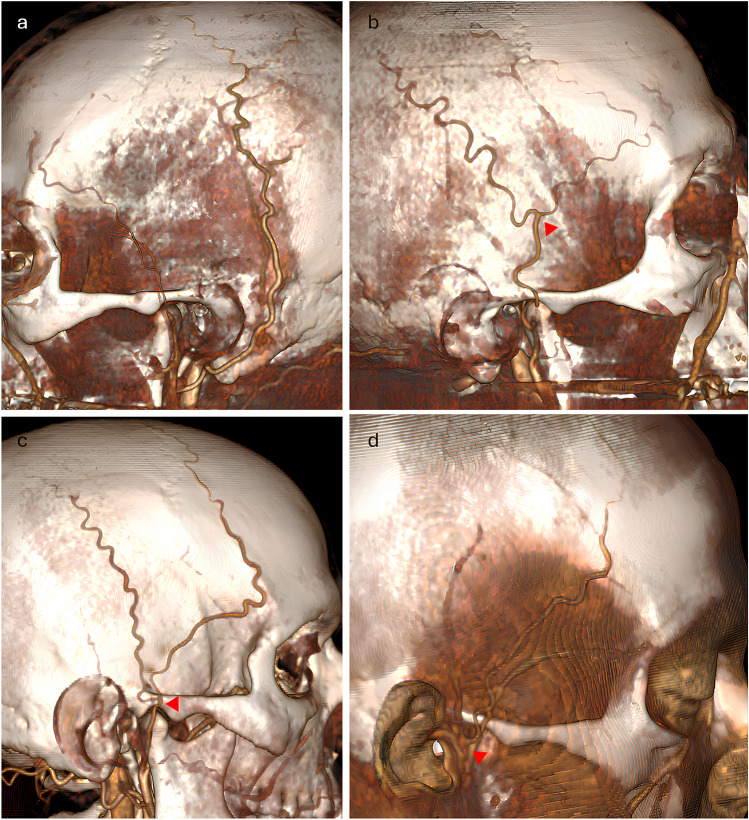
Location of the bifurcation point of STA (red arrowhead) relative to the ZA on three-dimensional volume rendering computed tomography angiography images. a Absent: No bifurcation. b Above: Located above the ZA. c Over: Located on the ZA. d Below: Located below the ZA.
